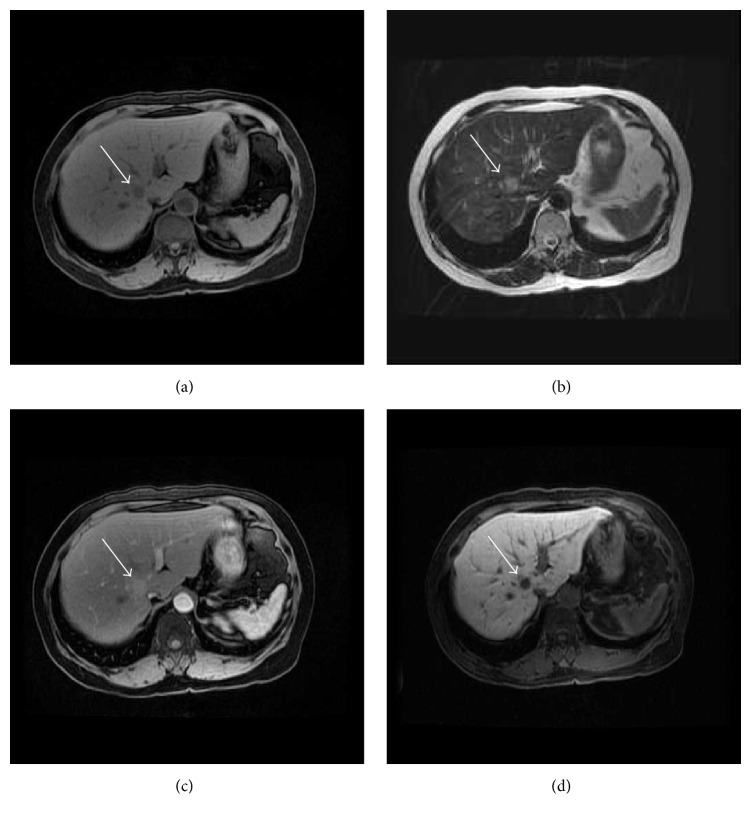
Magnetic resonance imaging. T1-weighted fat suppressed imaging showed low intensity at the tumor of S7 (arrow) (a). T2-weighted imaging showed high intensity at the tumor (b). In Gd-EOB-DTPA enhanced MRI, the tumor was enhanced in the arterial phase (c), and there was low signal intensity in the hepatobiliary phase (d).
